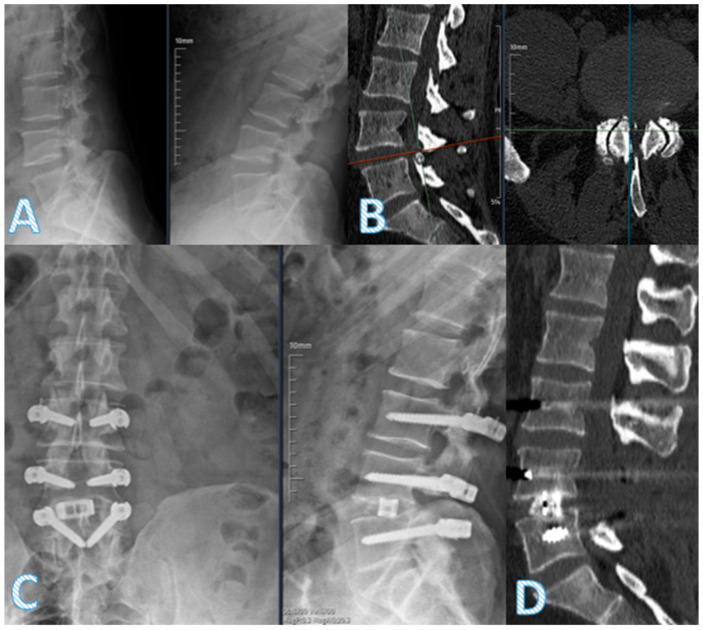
A 52-year-old female patient presented with severe low back pain (VAS 9), bilateral leg pain (VAS 7), neurogenic claudication at 50 m, and urge incontinence. Preoperative dynamic lateral lumbar X-rays in flexion and extension (A) and CT images (B) show advanced degeneration and instability at the L4–5 level with marked disk space narrowing. Postoperative anteroposterior and lateral lumbar radiographs (C) and sagittal CT (D) demonstrate successful decompression and stabilization following L4–5 transforaminal lumbar interbody fusion (TLIF) using a PEEK rod and interbody cage.
